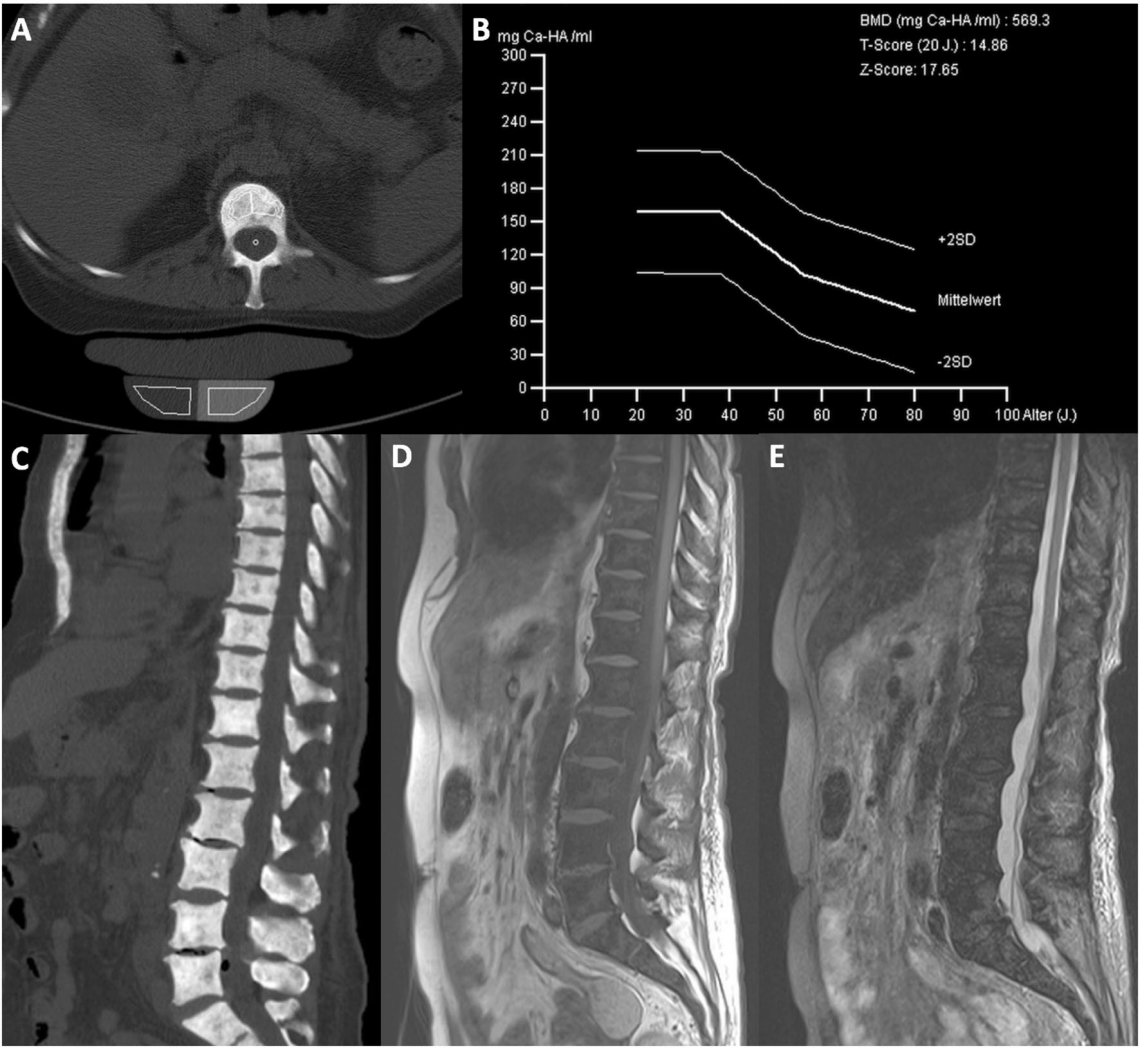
a Computed tomography (CT) scan of L1 and b bone mineral density (BMD) in a 77-year-old male patient with advanced systemic mastocytosis (AdvSM). Severe osteosclerosis is reflected in the *Z* score of 17.65 and in the corresponding CT scan of the spine (c). Also, magnetic resonance imaging (MRI) images of the spine showed diffuse osteosclerosis with a very low signal both in T1-weighted (d) and turbo inversion recovery magnitude (TIRM)-sequences (e)
